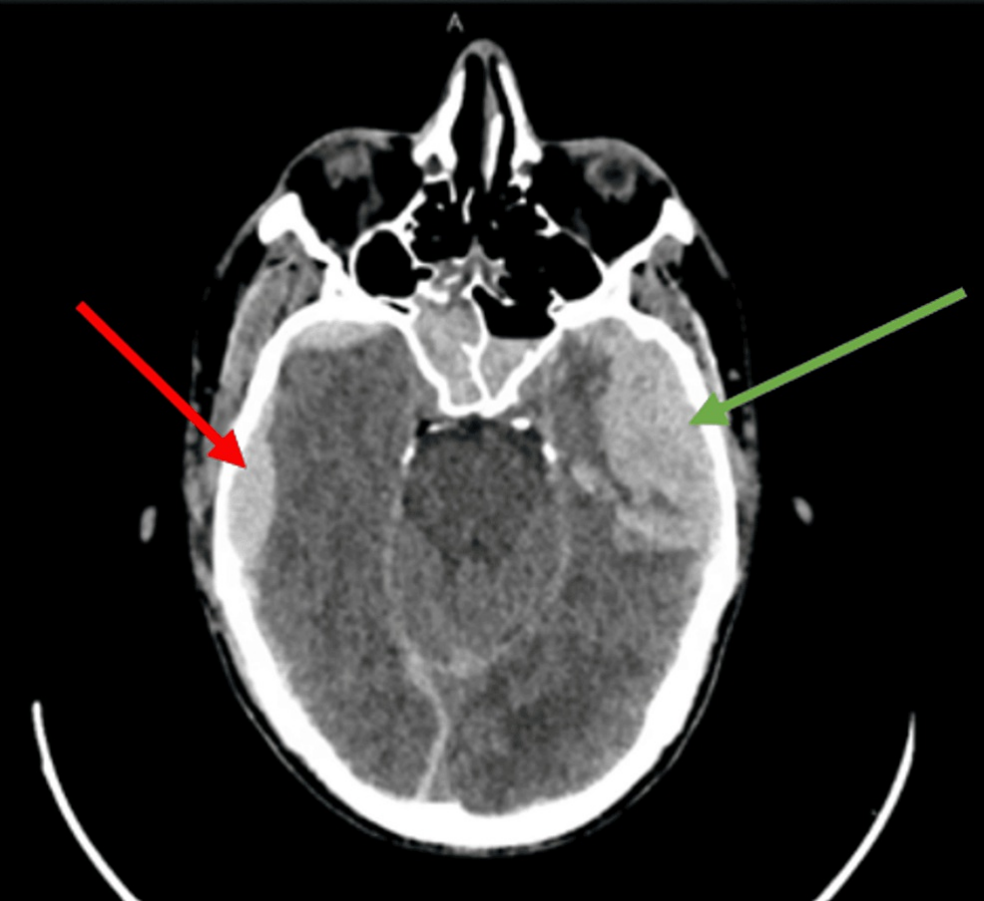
Head CT demonstrating right-sided epidural hematoma (red arrow) and left subdural hematoma (green arrow)
